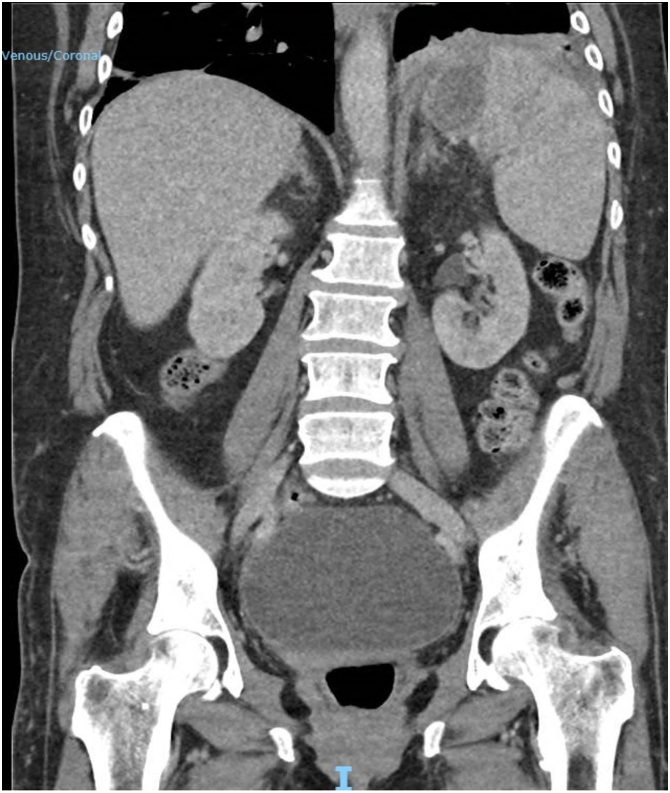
Abdominal computed tomography showing a coronal view of the splenic abscess and incidental large ovarian cyst.
